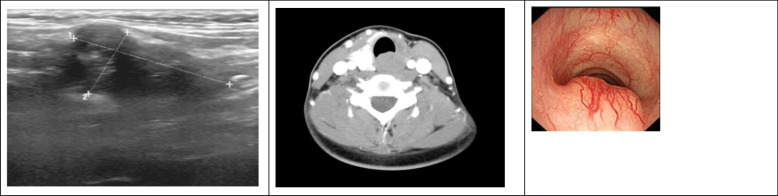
Pre-operative images, endoscopy and FNA performed before recurrent surgery. a Ultrasound located a hypoechoic mass on the left thyroid bed; b Neck computed tomography scanning showed that an unevenly-enhanced mass located posterior to the fibromuscular membrane of trachea; c Bronchoscopy showing that the mass exerted external pressure, causing stenosis of cervical trachea
